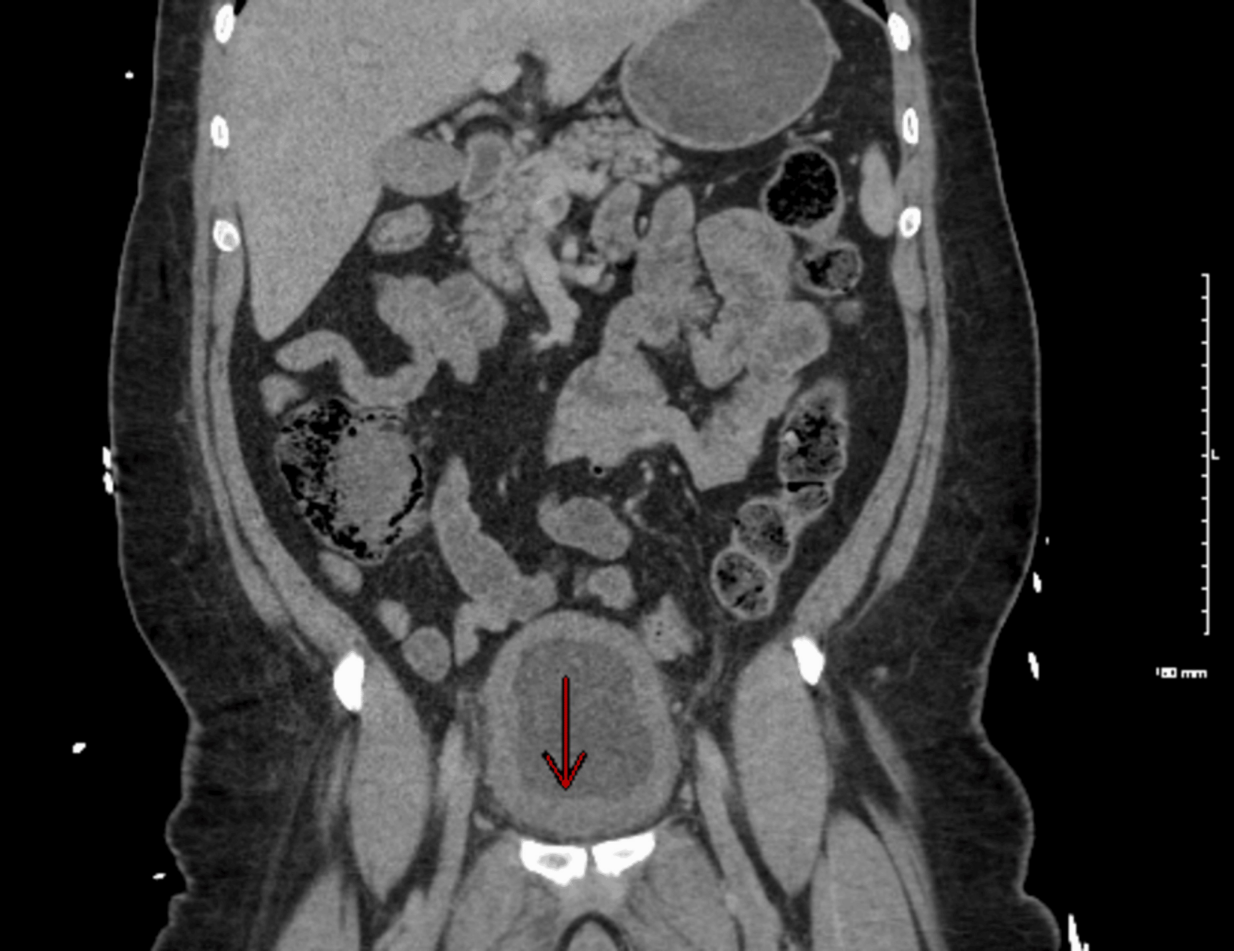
Bladder wall thickness CT scan of the abdomen and pelvis showing increased bladder wall thickness, pointed by a red arrow
